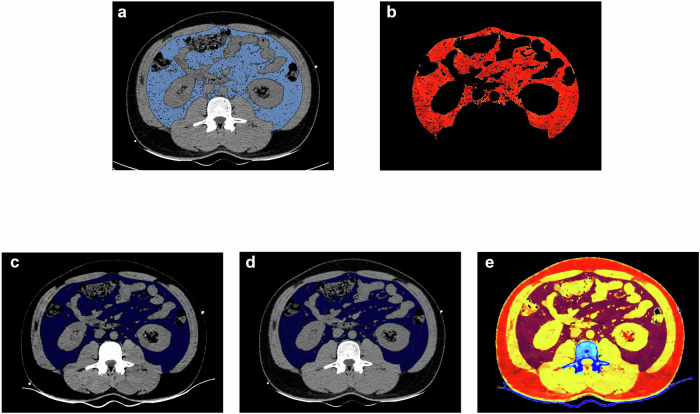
Method for measuring DECT-VAT parameters on abdominal computed tomography images at the 3rd lumbar vertebra in a case example of a 40-year-old female patient with MetS. a, b Under the conventional 120-kVp image, the visceral fat threshold was set to −190 to −30 HU, and the software automatically outlines the visceral fat tissue (a blue area; b red area), CT_poly_ = −111.3 HU, VAT = 106.5 cm^2^. c, d Virtual monochromatic imaging at 40 and 70 keV. The dark blue area is the outlined visceral fat, CT_40keV_ = −193.2 HU, CT_70keV_ = −109.8 HU. e The effective atomic number imaging pseudo-color map for the same case, Eff-Z = 5.77. DECT, dual-energy computed tomography; VAT, visceral adipose tissue
